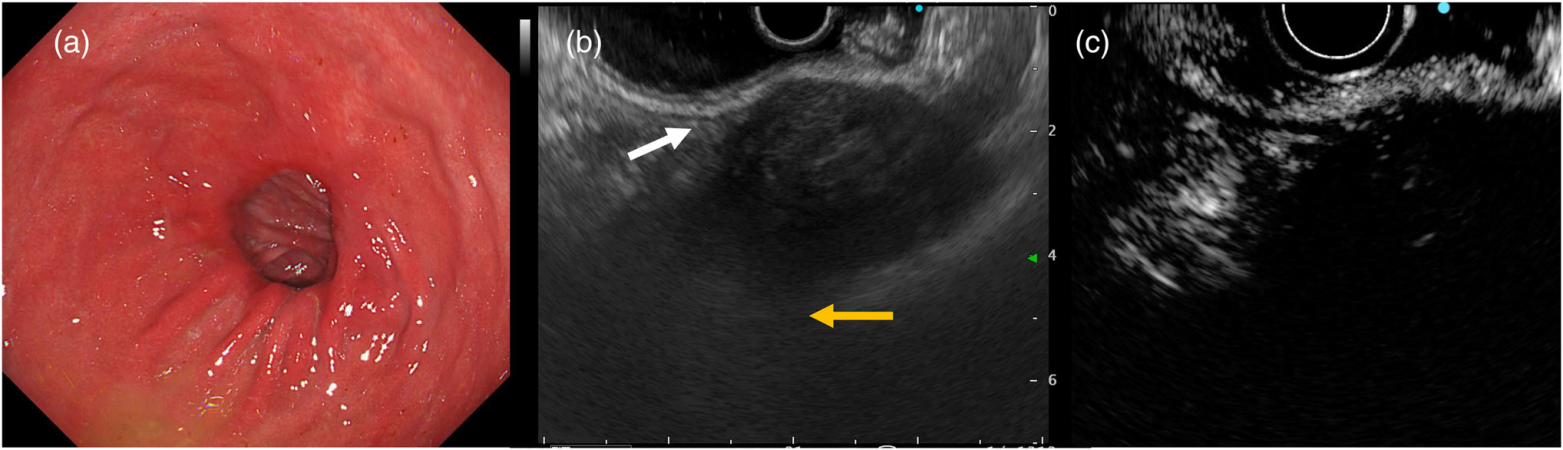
White light imaging (a): After pyloric gastrectomy and Billroth 1 method reconstruction. There was no obvious mass in the gastric lumen; Endoscopic ultrasound (b): Post‐acoustic shadow with low‐echoic mass. Post‐acoustic shadow (yellow arrow); Tumors appear to be in continuity with the gastric wall (white arrow); Contrast‐enhanced endoscopic ultrasound (c): No influx of perflubutane into the mass.
